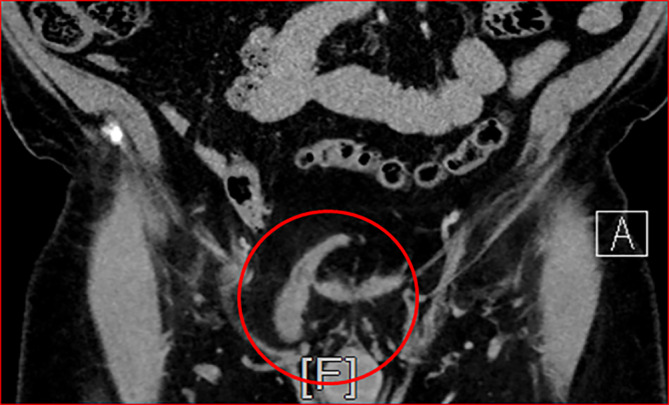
CT – Koronarscan (*roter Kreis*: umfährt die Ausdehnung der Inguinalhernie rechts mit Harnblasendivertikel im Bruchsack)
